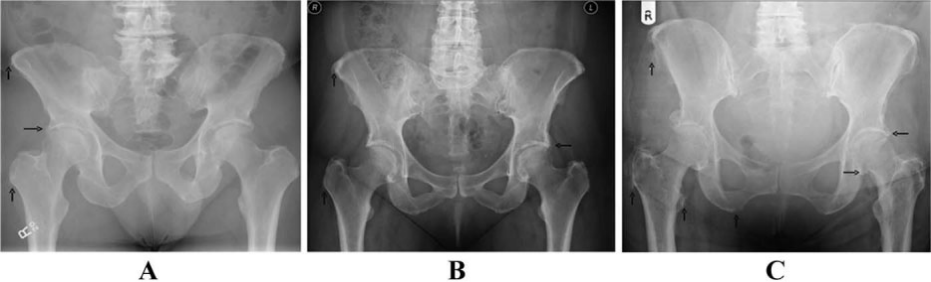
Pelvic radiographs obtained from our enthesophyte atlas, showing examples of mild enthesophytes (grade 1), characterized by subtle new bone formation at the anterior superior iliac spine (ASIS) and greater trochanter (vertical arrows) (A), moderate enthesophytes (grade 2), characterized by new bone formation mainly at the ASIS and greater trochanter (B), and florid enthesophytes (grade 3), characterized by marked new bone formation around the ASIS, iliac crests, greater and lesser trochanters, and, to a lesser extent, the ischial tuberosity (C). Horizontal arrows indicate the presence of osteophytes at the superior acetabular margin in A–C and at the left medial femur in C. Example images were made available as full-screen digital images to readers during scoring.
